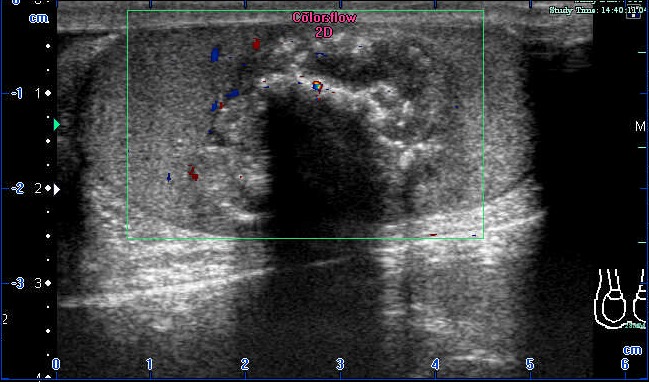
Scrotal ultrasound. The left testicular mass demonstrated blood flow in color Doppler sonography.
